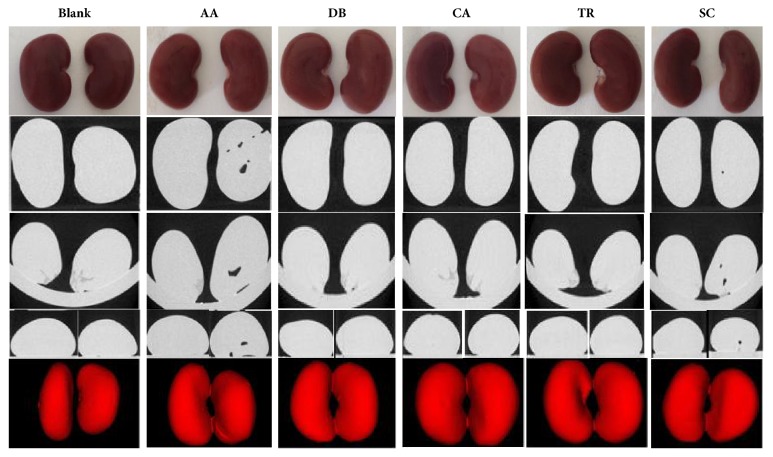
Photographs and micro-computed tomography scans showing the effects of nephrotoxic agents including aristolochic acid (AA), diosbulbin B (DB), cantharidin (CA), triptolide (TR), and sophocarpine (SC) on kidney shape, symmetry, and structure.
